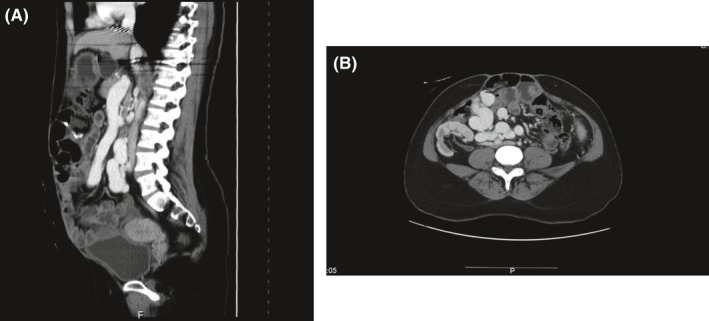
A, B, Computed tomography showing midline fascial defect
